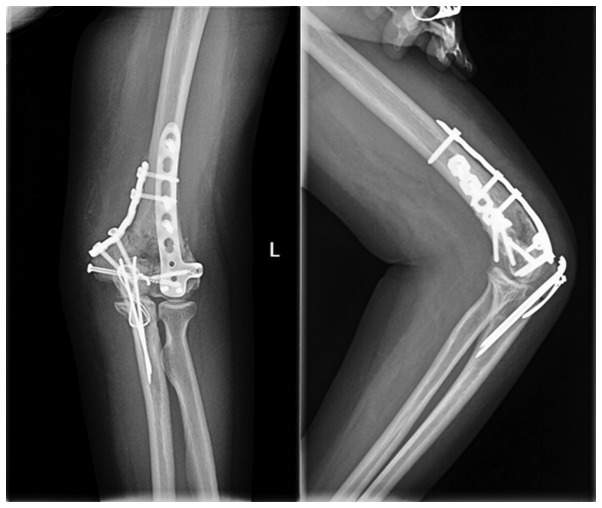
Postoperative radiographs following perpendicular plating fixation for distal humerus fractures.
